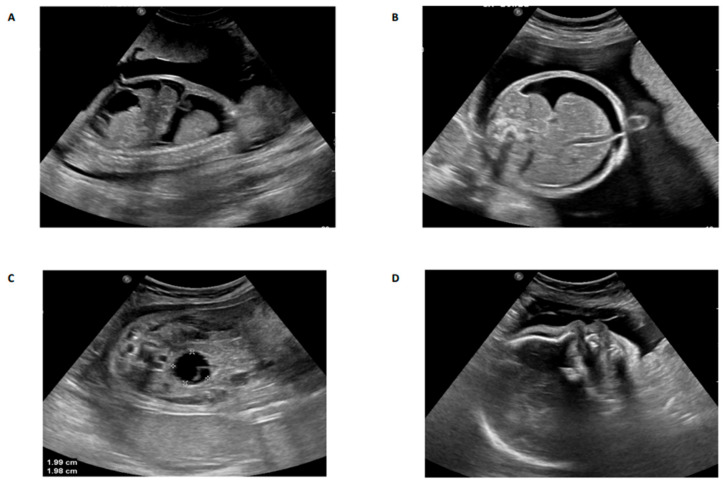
Antenatal ultrasound images of affected cases in family 1 (A,B) and family 2 (C,D). (A) Sagittal view of the fetal thorax and abdomen at 26 weeks of gestation, showing severe pleural effusion and ascites; (B) A transverse view of the fetal abdomen at 26 weeks of gestation showing ascites; (C) Coronal view of the fetal abdomen and pelvis at 29 weeks of gestation showing a well circumscribed hypoechoic area in the fetal abdomen which may represent an abdominal cyst with unknown origin; (D) Sagittal view of the fetal face at 29 weeks of gestation showing an abnormal profile with micrognathia.
